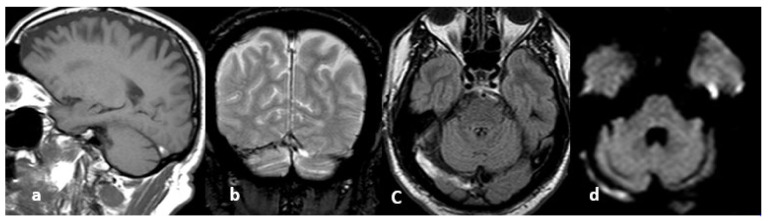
From left to right, spontaneous signal of the right lateral sinus suggesting thrombosis: (a) T1 hypersignal, (b) T2* hyposignal, (c) FLAIR hypersignal, (d) diffusion-weighted imaging hypersignal.
